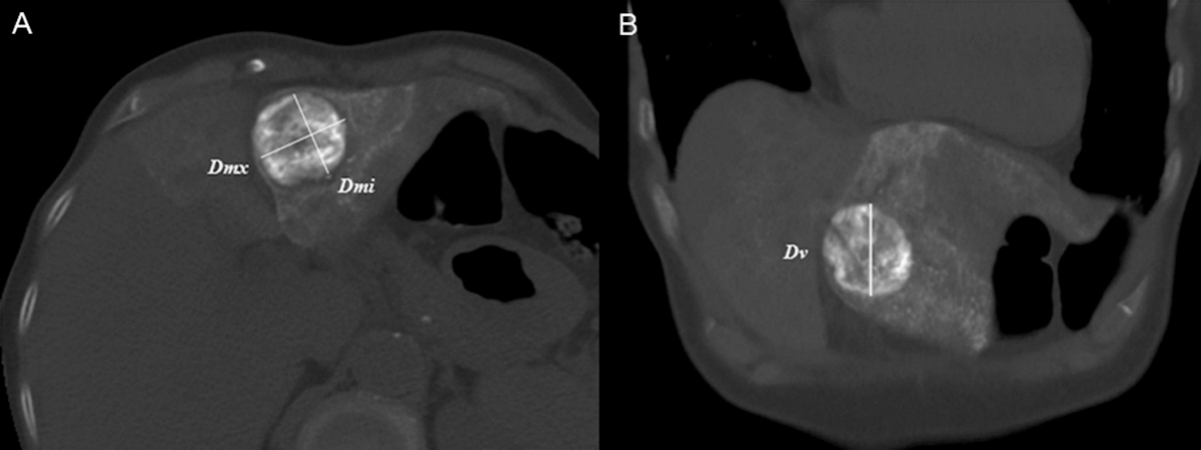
Measurement of three-dimensional (3-D) tumor diameters. Axial (a) and coronal (b) CT images best depicting index tumor serving in 3-D diameter measurements as follows: maximum diameter (Dmx) and perpendicular minimum diameter (Dmi) in axial image (a), and vertical diameter (Dv) in coronal image (b). Note: a preset bone window setting (width, 3000 HU; level, 500 HU) is applied to avoid beam-hardening artifact due to iodized oil retention by index tumors or surrounding parenchyma.
